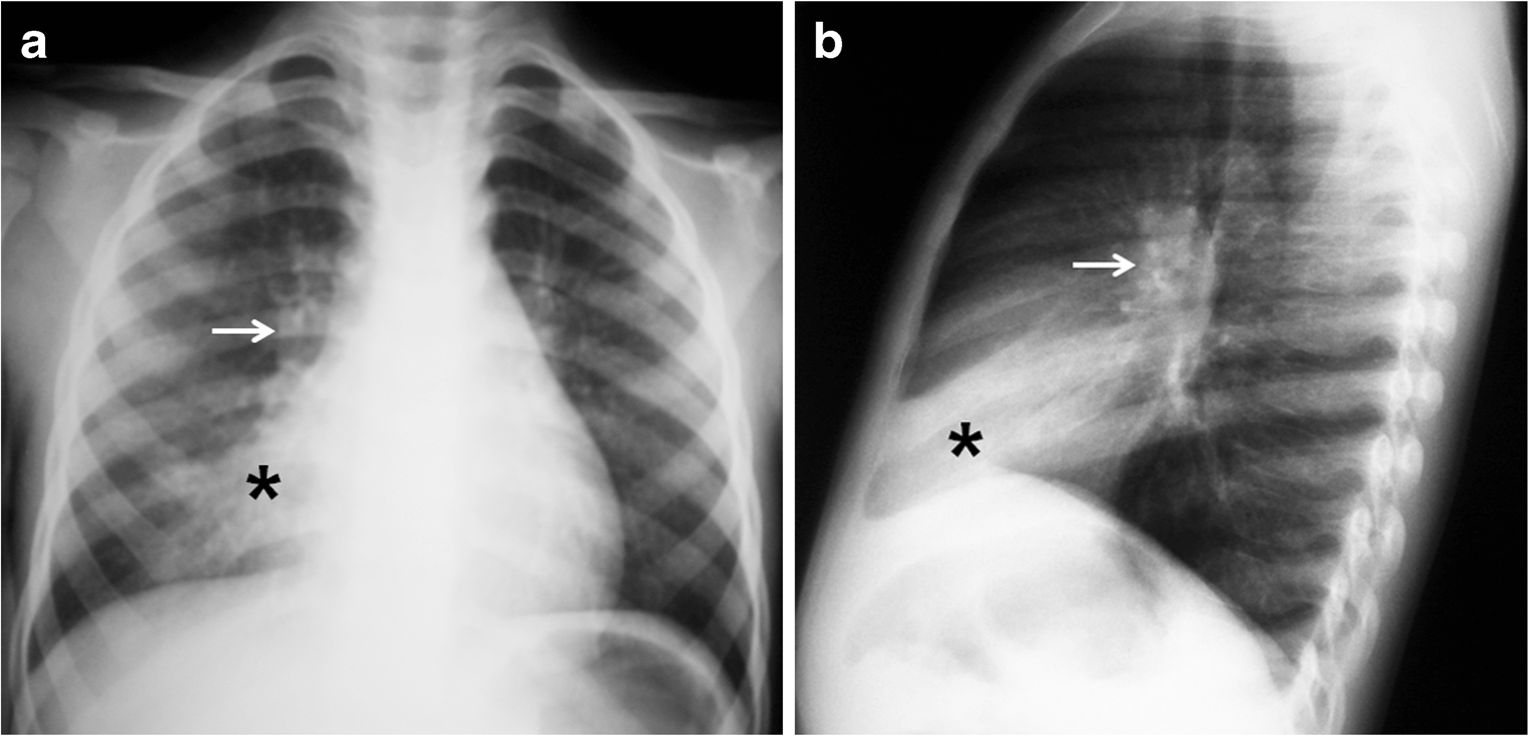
Chest radiographs in a 5-year-old girl with primary tuberculous disease. a, b Anteroposterior (a) and lateral (b) views show a middle lobe opacity (*asterisk*) with right hilar lymphadenopathy (*arrow*)
